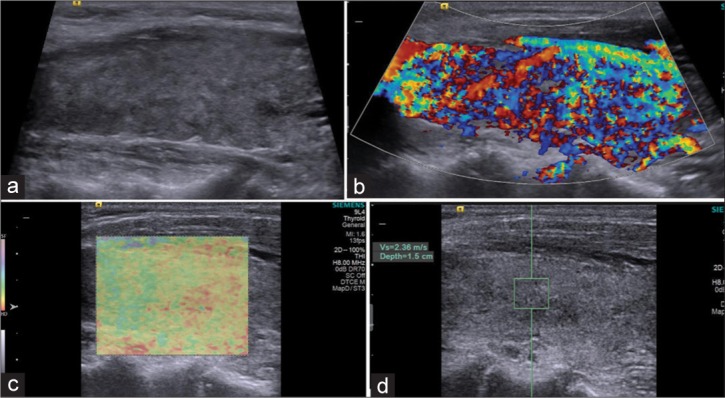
Patient with Graves’ disease: (a) Grayscale ultrasound showing hypoechoic echopattern. (b) Color Doppler: Type III pattern (thyroid inferno). (c) Elstogram reveals type II pattern. (d) Shear wave velocity 2.36 m/s (depth 1.5 cm).
